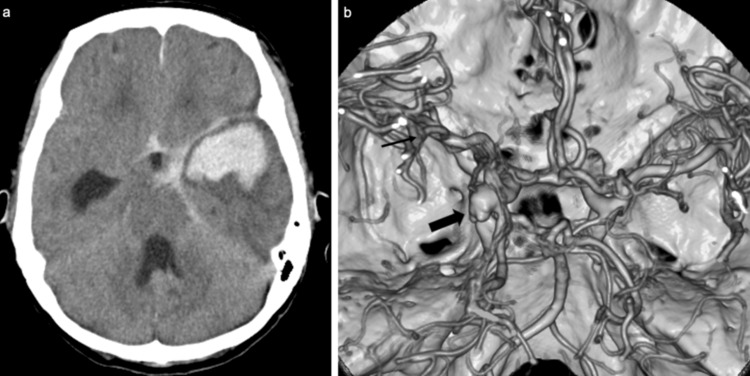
Preoperative CT and CTA images (a) Axial CT scan showing diffuse SAH and left intratemporal cerebral hemorrhage. (b) CTA indicating the ruptured aneurysm at the left internal carotid-posterior communicating artery bifurcation (maximum diameter, 6.7 mm; arrow). CTA, CT angiography; SAH, subarachnoid hemorrhage
